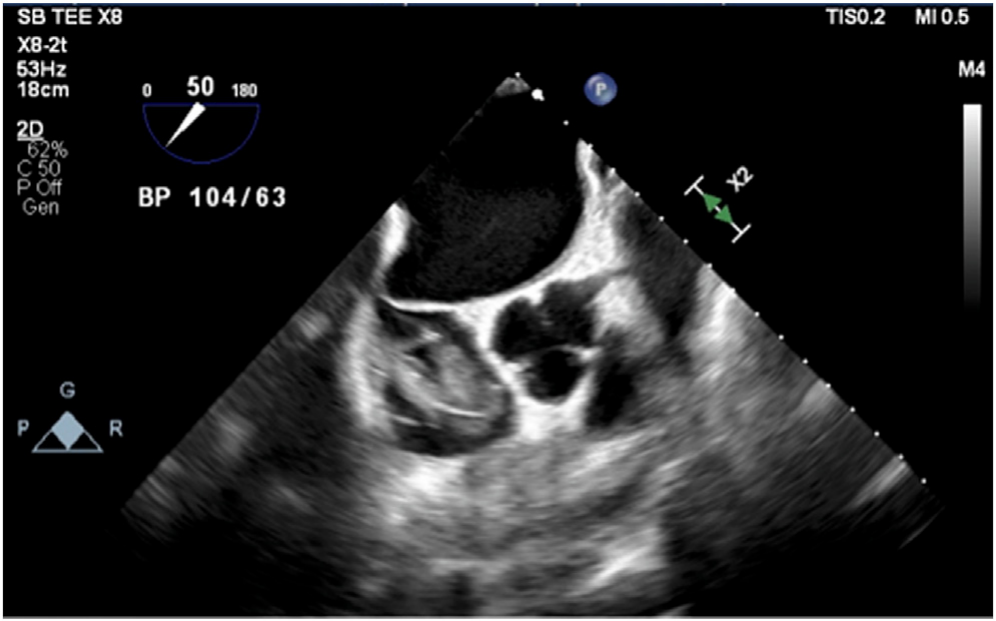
Transesophageal echocardiogram demonstrating a right atrial lead-associated thrombus.
